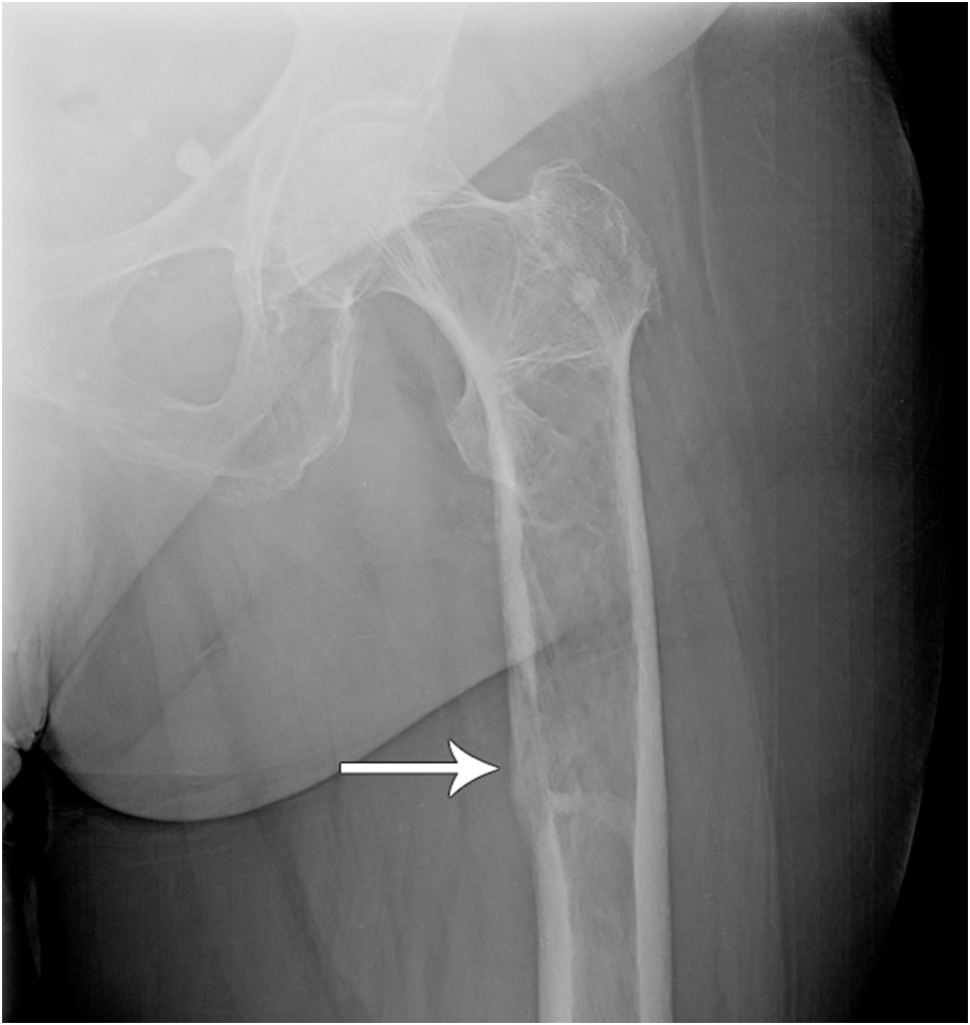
AP radiograph of the left hip demonstrates a hemangioma that is lytic, peritrochanteric, and involves greater than 2/3 of the bone width, including medial cortical involvement (*arrow*). Even without information regarding pain, this lesion would have a minimum Mirels’ score of 9 and therefore should undergo prophylactic fixation
